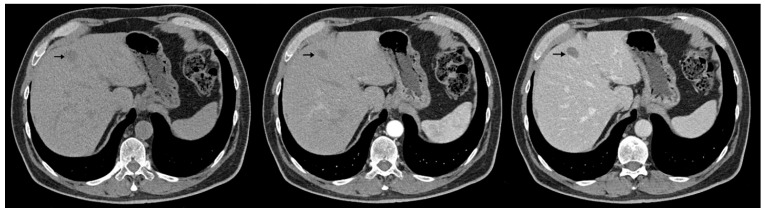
Contrast-enhanced abdominal CT (axial, non-contrast phase—left, arterial phase—center, and portal venous phase—right) shows liver sections with hypodense nodule in IVa hepatic segment exhibiting arterial-phase enhancement similar to background parenchyma and washout on the portal venous phase, measuring 1–3 cm (arrows).
